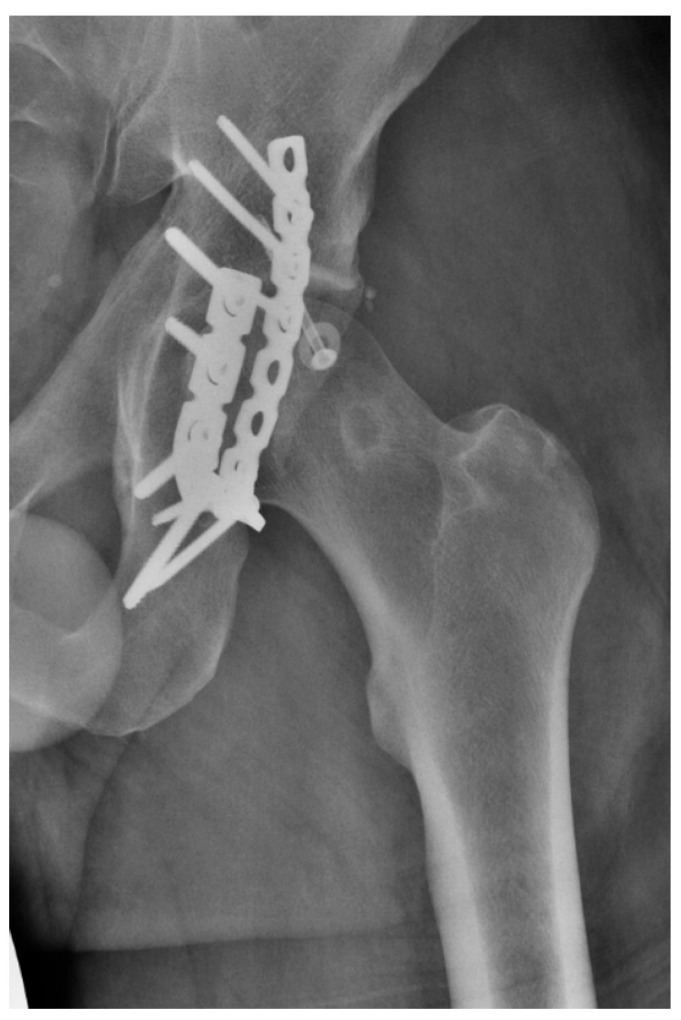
AP X-ray of a pelvic overview 6 weeks postoperatively after open retrieval of the free joint body and stabilization of the acetabular fracture using a two-plate osteosynthesis.
